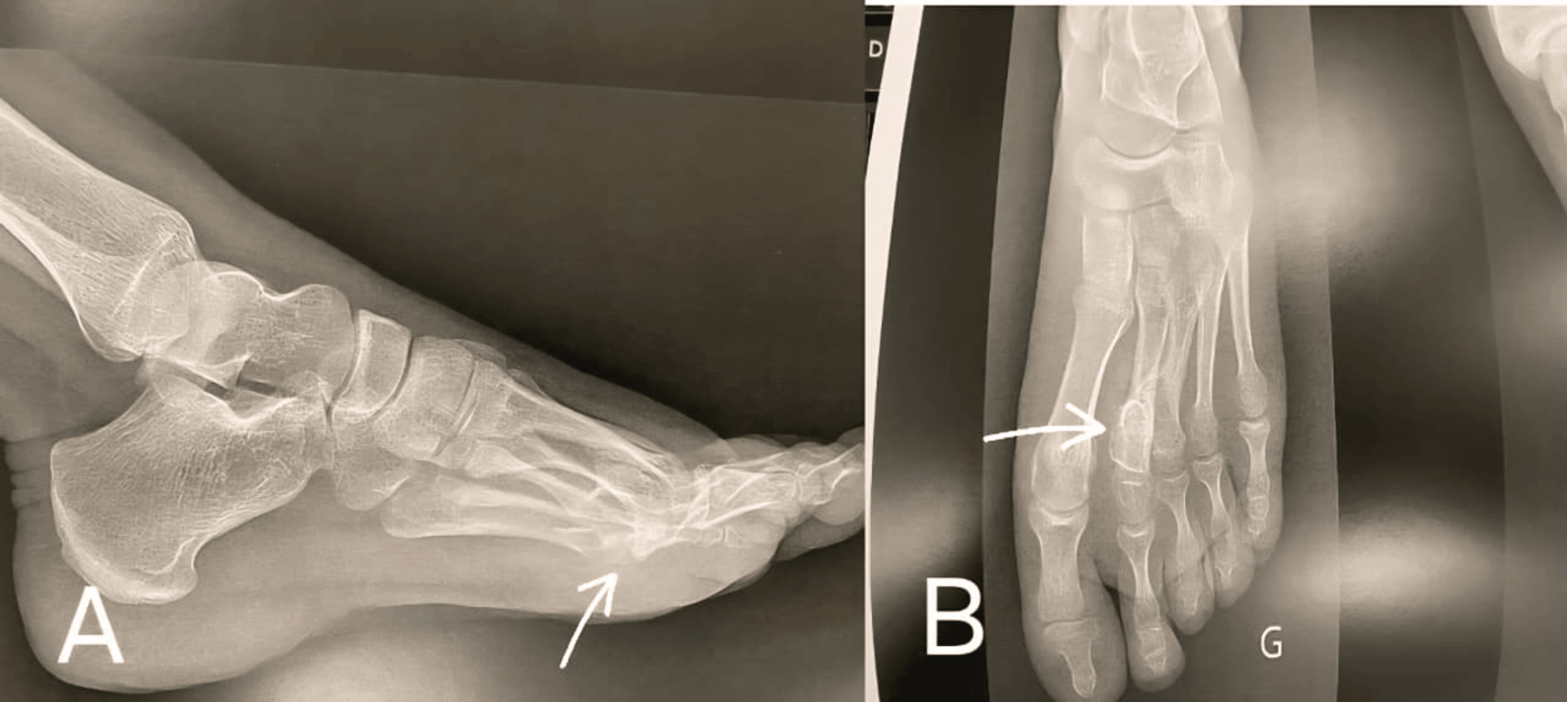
Lateral (A) and anteroposterior (B) radiographs of the left foot at one year postoperatively. White arrows indicates the location of the old osteochondroma.
